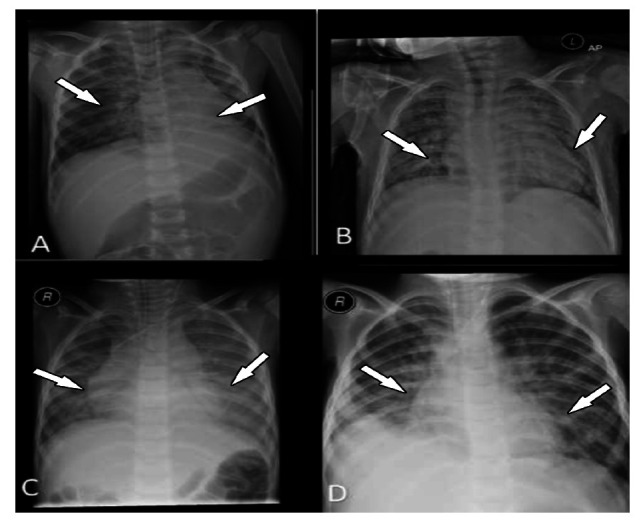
Chest radiographs of 4 pediatric patients with positive COVID-19 results. A) A 3-year-old male patient with COVID-19; initial chest radiography after intubation shows bilateral central ground-glass opacities with left lower lobe consolidation. B) An 8-year-old male patient with underlying severe bronchopulmonary dysplasia was admitted with COVID-19; chest radiography showed bilateral ground-glass opacities (more on the left). C) Chest radiography of a 4-year-old patient showing bilateral ground-glass opacity. D) Chest radiography of a 5-year-old showing bilateral consolidation with right lower lobe localization. This patient eventually died.
